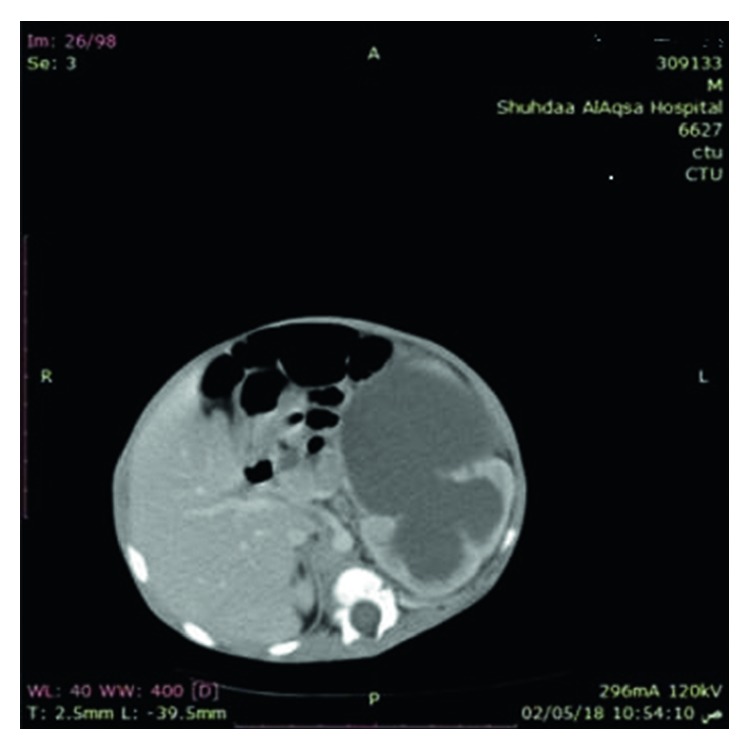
Abdominal CT showed left-sided pelvic-ureteral junction severe stenosis.
